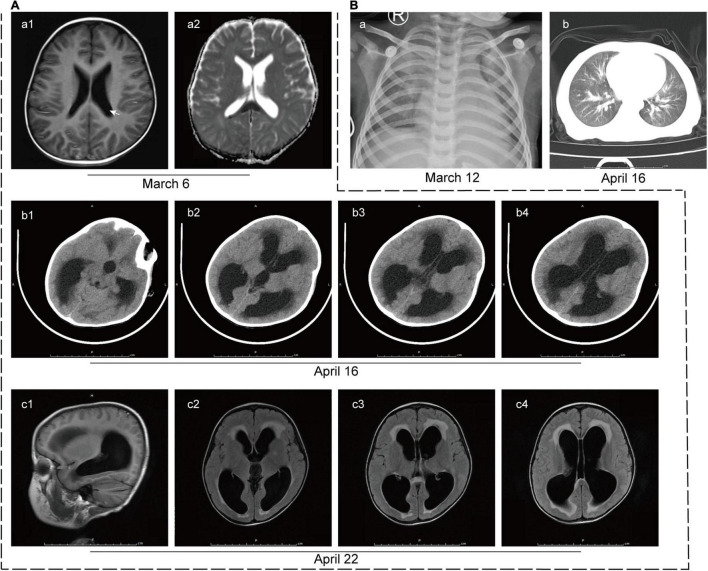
Imaging tests of the patient. (A) Imaging tests of the brain. (a) Brain diffusion-weighted MRI on 6 March 2020 revealed multiple intracranial abnormal signals with the slightly enlarged posterior horn of the lateral ventricle (white arrow). (b) CT scan of the brain on 16 April 2020 showed hydrocephalus and interstitial edema of white matter. (c) T2-Flair MRI images of the brain on 22 April 2020 showed multiple patchy shadows in the splenium of the corpus callosum, frontal lobe, and left basal ganglia, suggesting infectious changes. Hydrocephalus and interstitial edema were also observed. (B) Imaging tests of the lung. (a) The initial lung X-ray image of the patient on 12 March 2020 showed infection in the bilateral lungs. (b) CT scan of the lung on 16 April 2020 revealed patchy shadows on bilateral lungs, suggesting infection.
